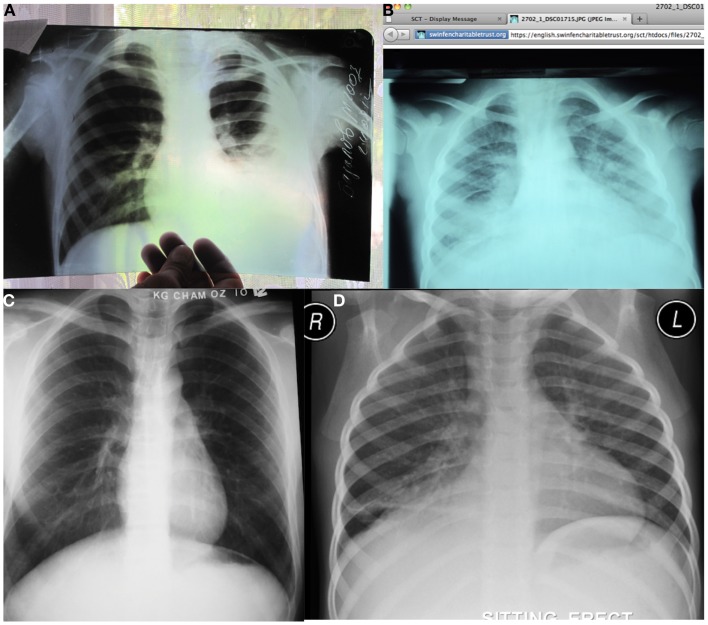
A variety of pediatric telereading referrals. (A) An X-ray referral from an MSF site in Tajikistan was received as a JPEG created by holding the hard copy X-ray up against a window and photographing it digitally. The curtains on the backdrop are seen through portions of the radiograph as a fine checker pattern. Although this was an inadequate X-ray referral the left-sided effusion is clearly visible. (B) Pediatric X-ray referral from the Collegium Telemedicum platform for MSF. The radiograph is of relatively poor quality as it is underinspired and the patient is markedly rotated to the left. In addition, the image has not been converted to gray scale. The right middle lobe air-space disease is visible but difficult to distinguish from density at the bases probably the result of poor inspiration. It is also difficult to comment on the presence of lymphadenopathy due to the vascular crowding, again due to under inspiration. (C) A radiograph referred from an MSF site in Cambodia is of higher quality, probably owing to the digital equipment available, which also avoids the quality losses from photographing or scanning hard copies. Note that positioning quality is improved with older patients but that on this occasion the right costophrenic angle has been cut off. (D) A digital X-ray referral from Khayelitsha District Hospital involves direct conversion of a DICOM image to JPEG for limiting file size and e-mailing through a control telereader to an expert pediatric radiologist. On this radiograph, a right lower lobe air-space disease process was reported but there were no features identified to suggest tuberculosis. The radiographic quality and labeling is excellent, as there are professionally trained radiographers working on-site.
